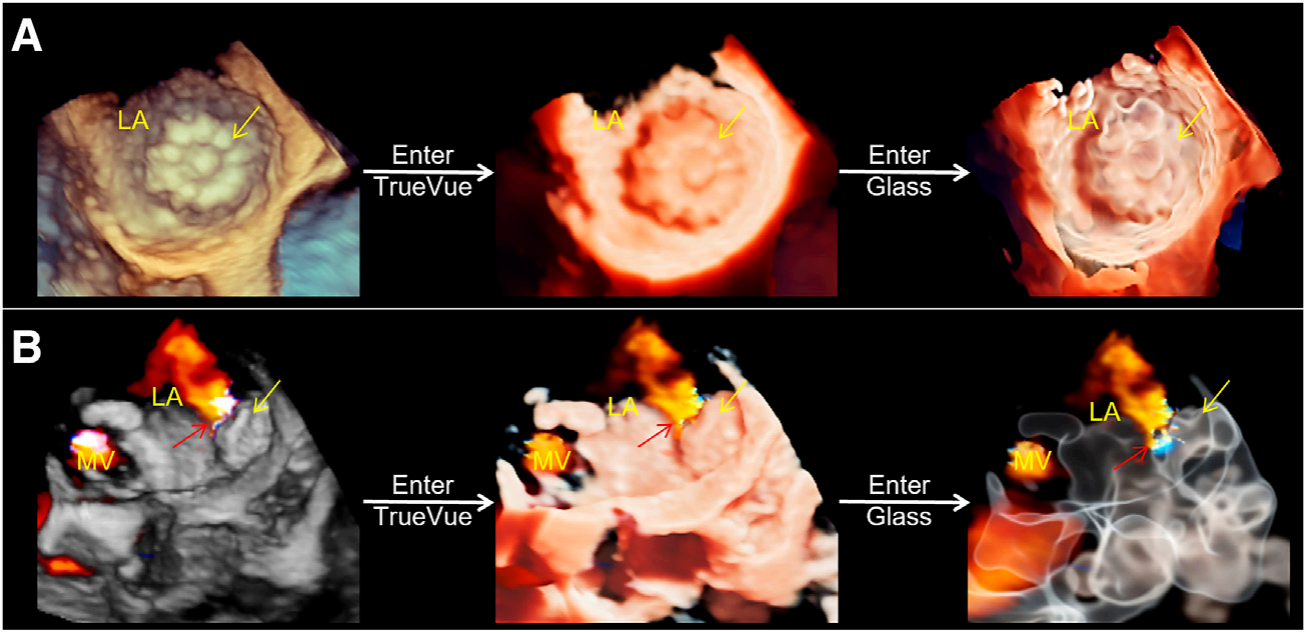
Three-dimensional transesophageal echocardiography plus color Doppler for outcome assessment after left atrial appendage occlusion. (A). From left to right, conventional 3D, TrueVue and TrueVue Glass direct views of the left atrium appendage occluder (yellow arrows) are displayed. (B). From left to right, conventional 3D, TrueVue and TrueVue Glass with color Doppler showing the residual shunt (red arrows) around the occluder (yellow arrows), among them, TrueVue Glass showing the origin and path of the shunt more entirely. LA, left atrium; MV, mitral valve.
